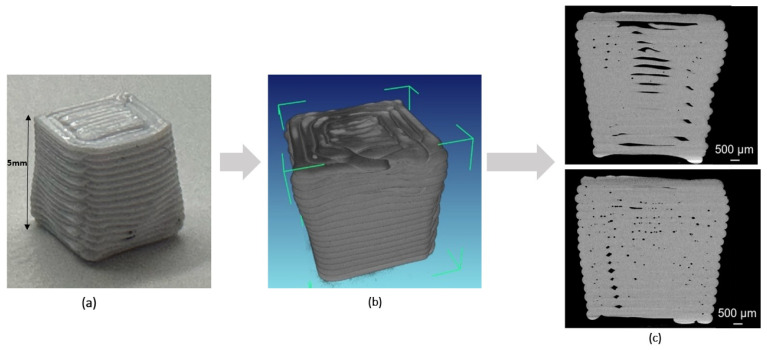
Process of image extraction: (a) 3D-printed cube, (b) digital file from the CT scan software, (c) generated 2D images of the cross-section along the z-axis.
